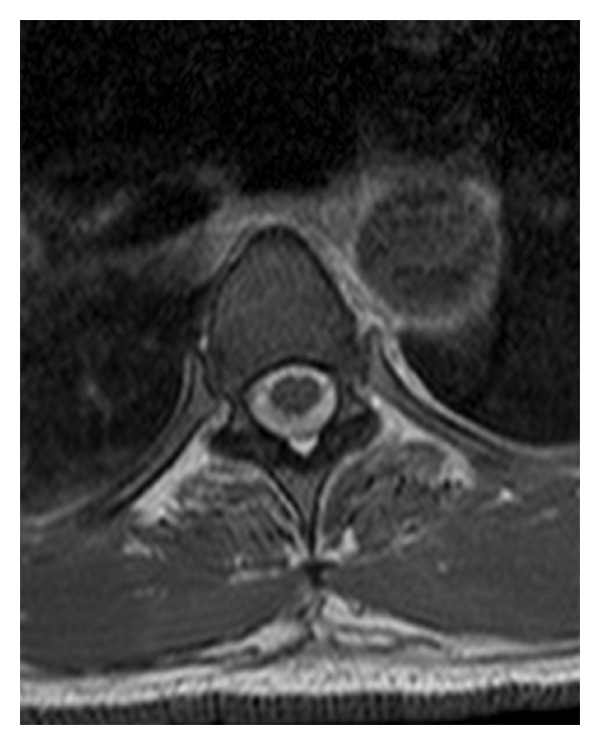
Axial T1-weighted dorsal MRI showing diffuse infiltration of the tumor.
